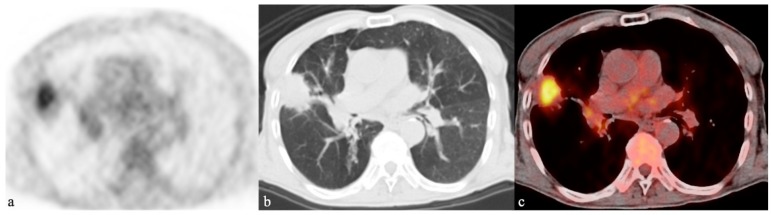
A 79-year-old male affected by pneumonia due to *Pseudomonas aeruginosa* who was examined by ^18^F-FDG-PET/CT. The ^18^F-FDG-PET (a), axial CT (b), and fused image (c) of the ^18^F-FDG-PET/CT scan demonstrate the pulmonary consolidation, with high tracer uptake, in the middle lobe due to the infection.
